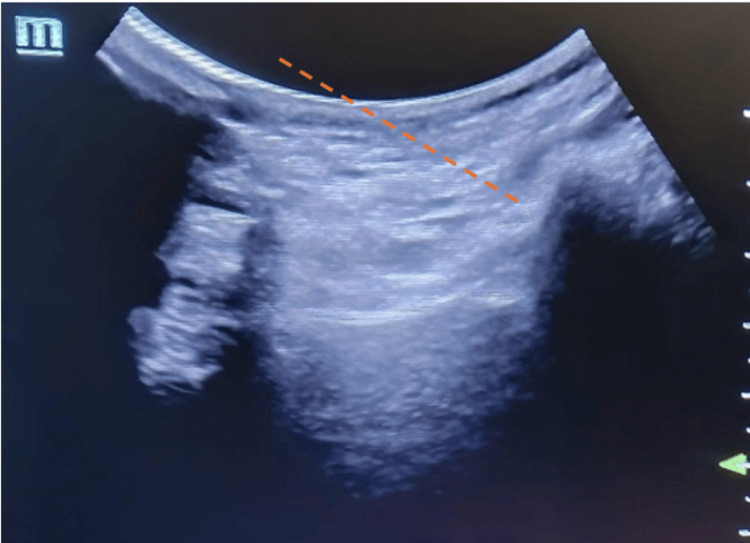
Ultrasound image of the sacroiliac joint The orange dashed line represents the path of the needle toward the sacroiliac joint
